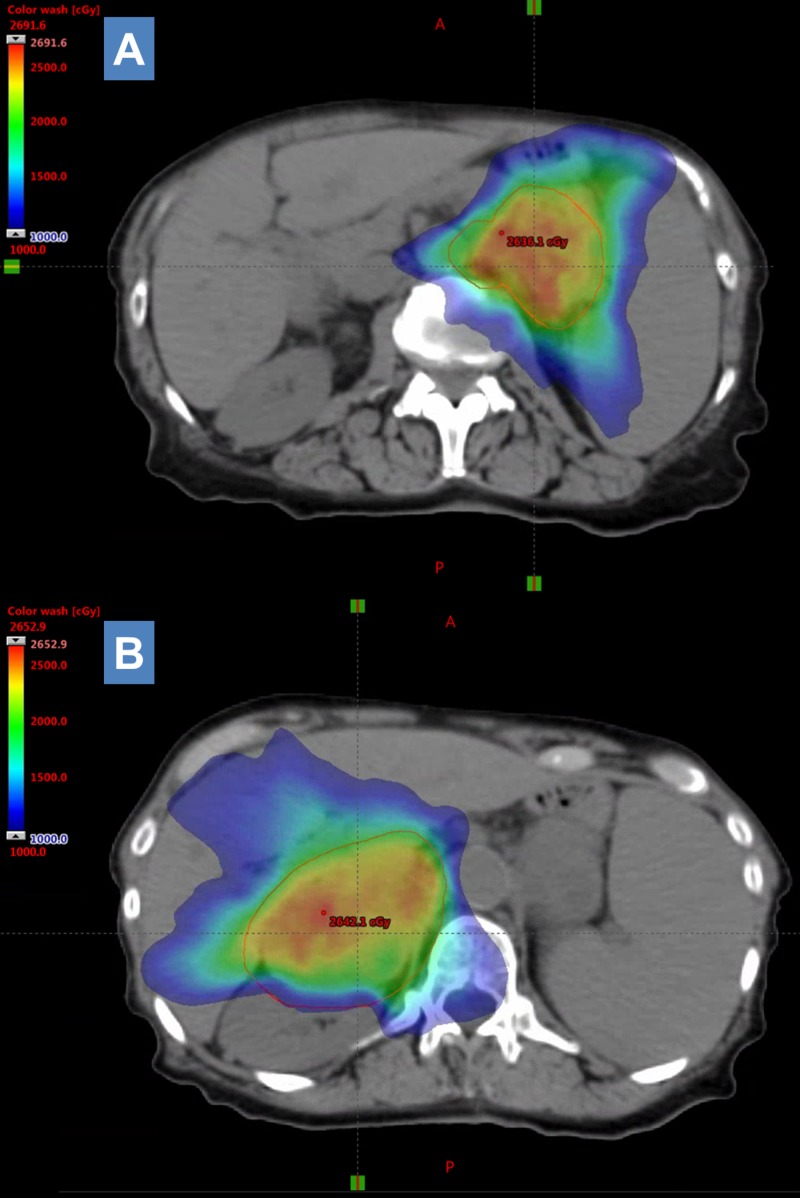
Stereotactic radiation treatment plan selected axial slices representing spatial distribution of dose. Left (A) and right (B) adrenal metastases were treated with stereotactic radiation to a total dose of 25 Gy in five fractions with steep dose drop-off as visualized by the continuous dose gradient overlaying treatment planning computed tomography (CT) scan. The left para-aortic lymph node was included in the left adrenal mass target volume.
